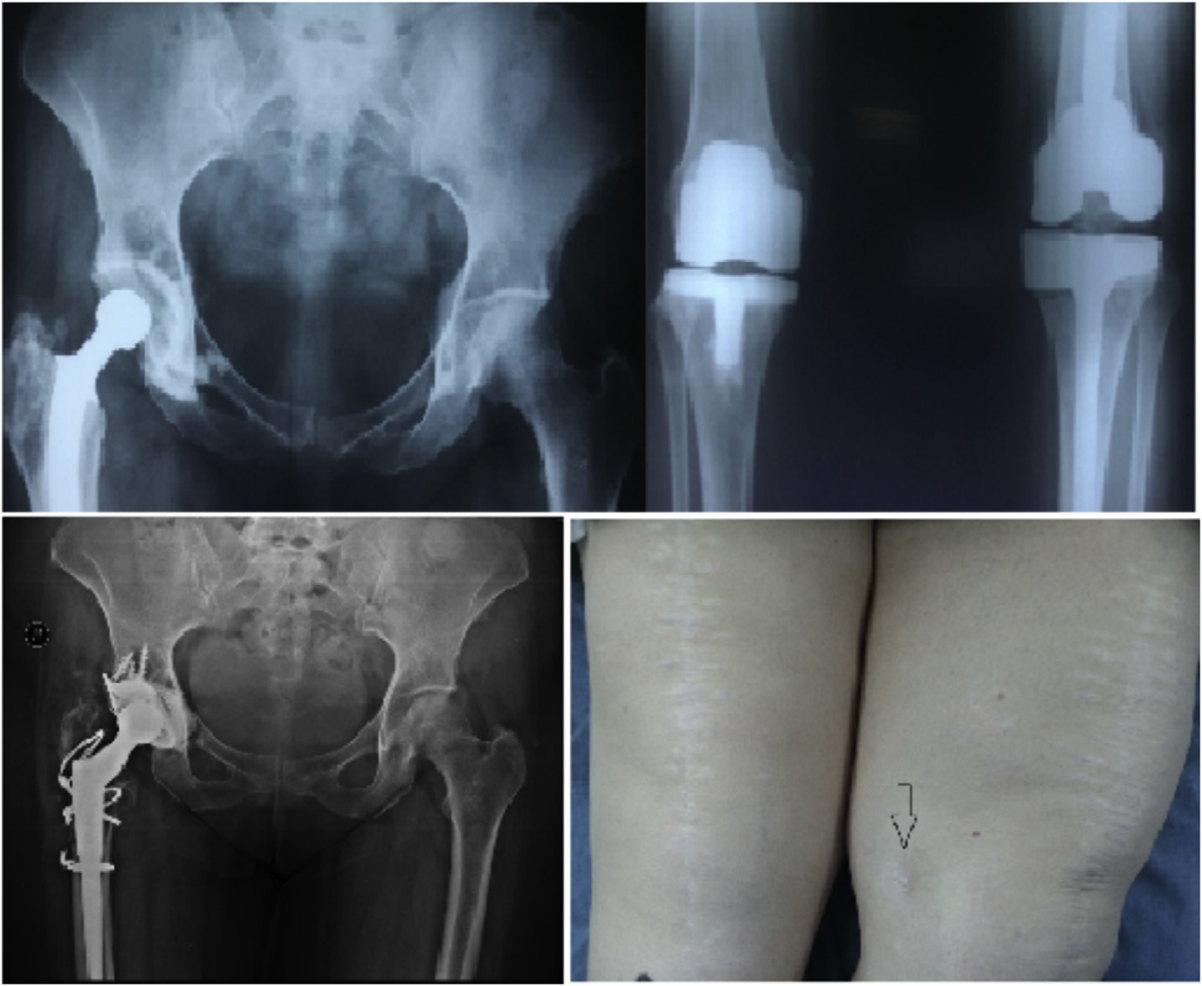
Preoperative X-ray image showing bilateral knee prostheses and right hip prosthesis and postoperative X-ray image of the revision hip prosthesis performed in the presence of a discharging sinus in the left knee (arrow).
